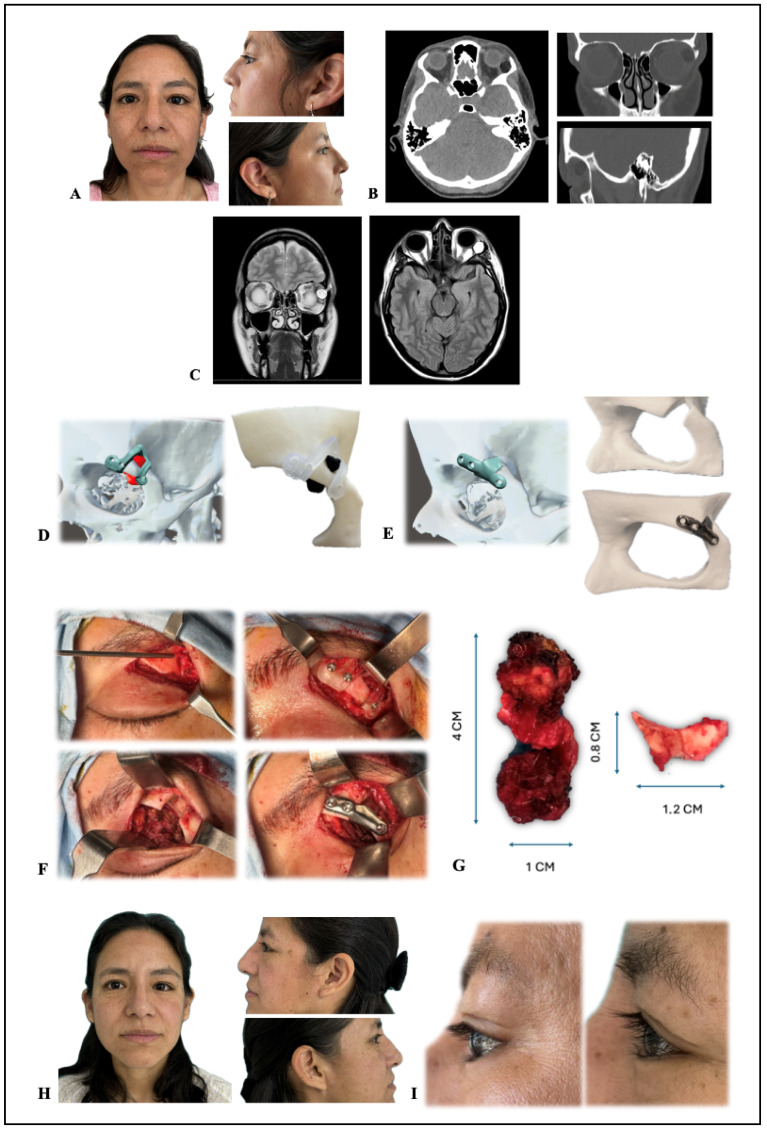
(A) Preoperative photos showing the asymmetry caused by the left-side proptosis. (B) CT showing a left superotemporal intra and extraconal lesion suggestive of dermoid cyst. (C) MRI confirming retroconal localization with cystic component (fluid–fluid level). (D) Stereolithographic orbit–tumor models and cutting guides (printed in Biomed Clear Resin). (E) PLA models, and a customized supraorbital titanium patient-specific implant (PSI). (F) Sequential surgical steps (to be viewed from upper left to right, then lower left to right): (1) upper eyelid crease incision and exposure; (2) fixation of biocompatible resin 3D cutting guide; (3) osteotomy and en bloc resection of the extraconal dermoid cyst; (4) reconstruction with customized supraorbital titanium PSI. (G) Osteotomized bone flap from the planned superolateral orbital roof window and microsurgical en bloc resection of the extraconal dermoid cyst. (H) Clinical images of the patient at 2-month postoperative follow-up. (I) Comparative pre- and 3-month postoperative view of the surgical area.
